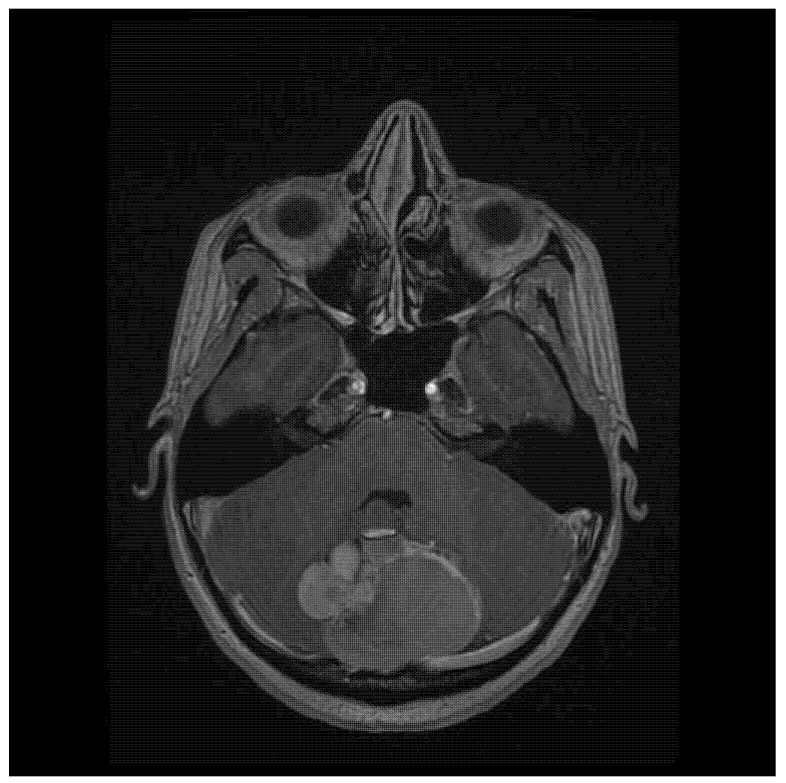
An axial contrast-enhanced T1-weighted image showing the tumor and the bone loss of the occipital bone.
